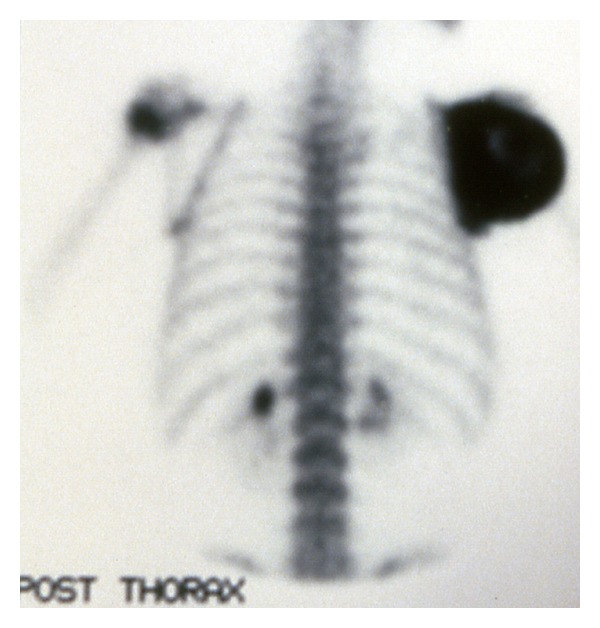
^99m^Tc-MDP bone scintigraphy. Posterior aspect.
